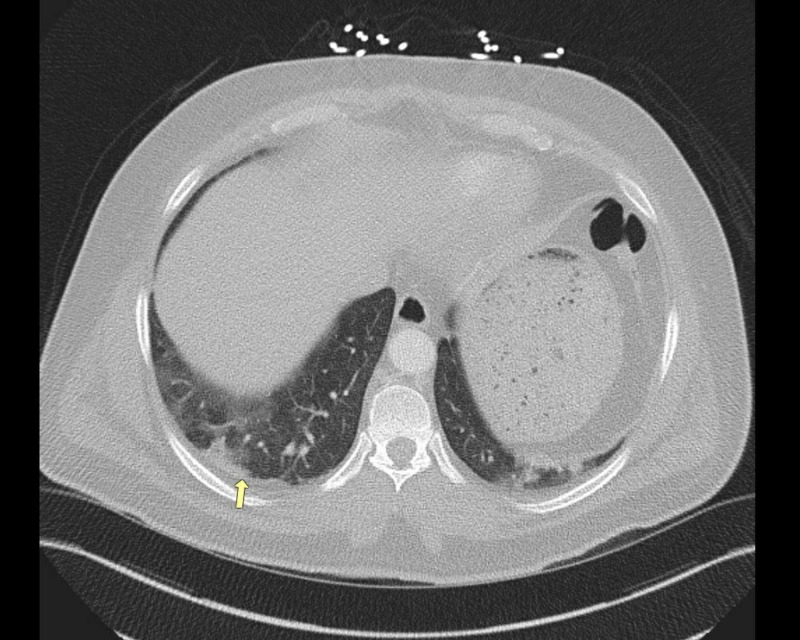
CT angiogram of the chest, with nodular opacities (arrow) CT: computed tomography
